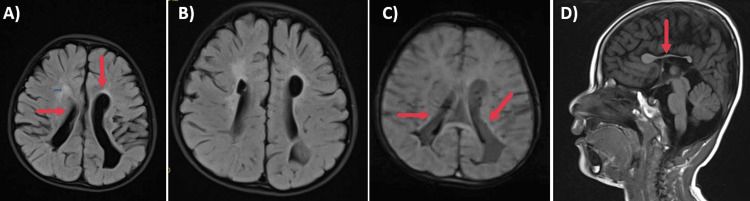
Magnetic resonance imaging (MRI) Brain A) MRI brain FLAIR axial section – showing asymmetric dilatation and squaring of occipital horns of lateral ventricles, hyperintensities along periventricular white matter and periventricular cysts. B) MRI brain FLAIR axial section – showing periventricular white matter hyperintensities and porencephalic cysts. C) MRI brain susceptibility-weighted image showing blooming with evidence of intraventricular bleed inside lateral ventricles. D) MRI brain - T1 sagittal section showing a dysplastic corpus callosum.
